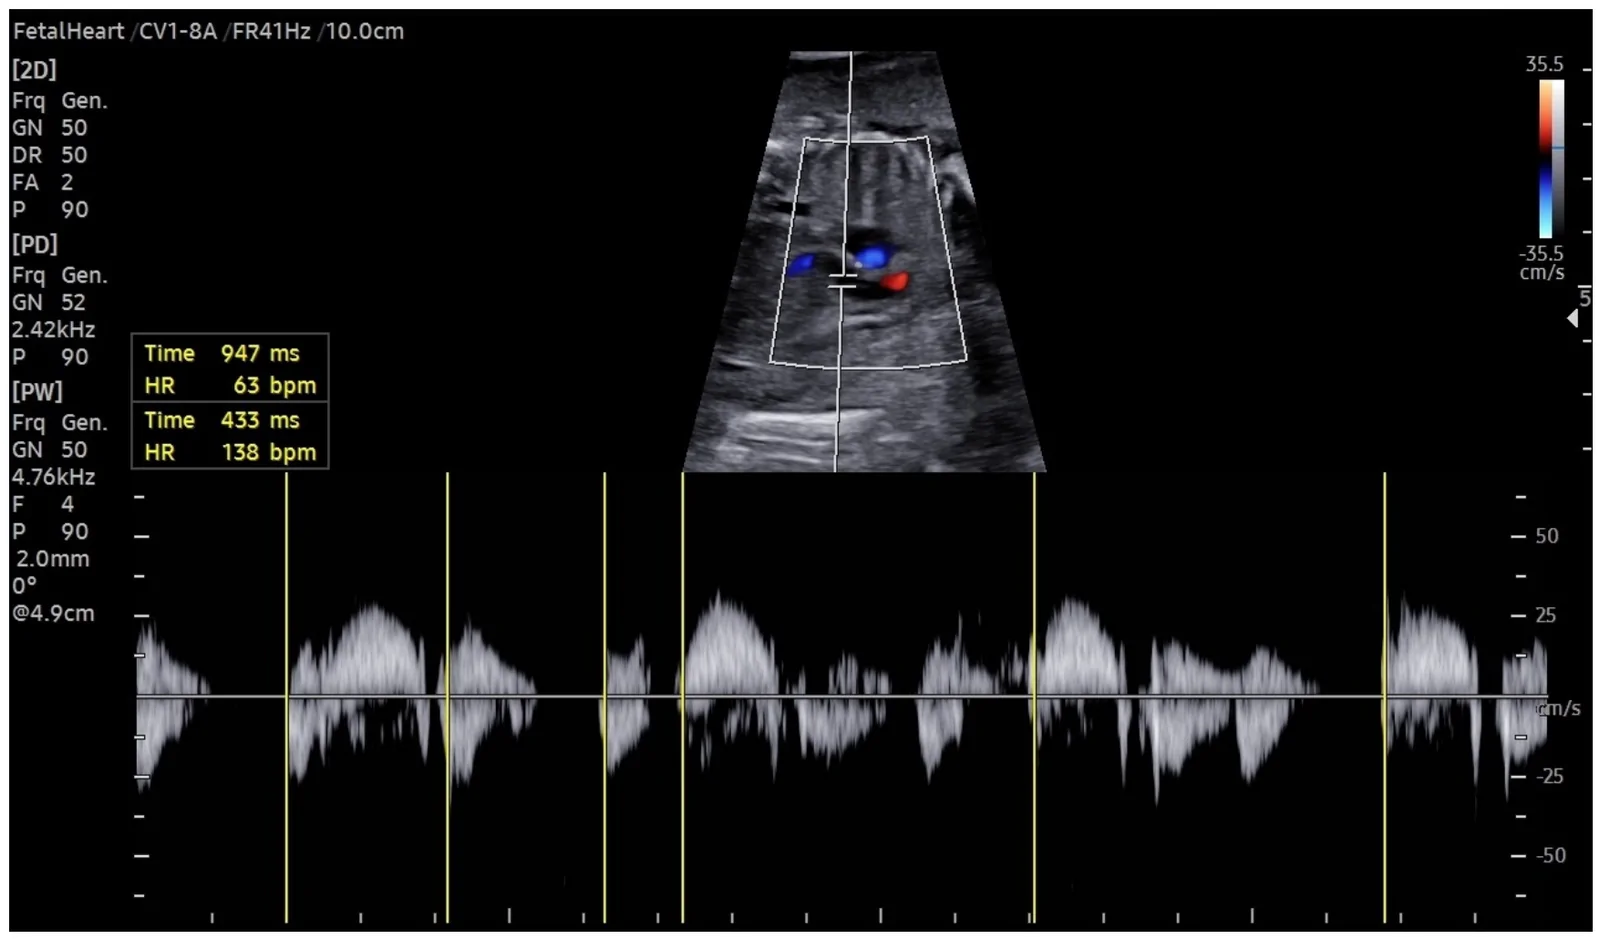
Representative echocardiographic image of the affected fetus, demonstrating the difference between atrial (138 bpm) and ventricular (63 bpm) pacing.
